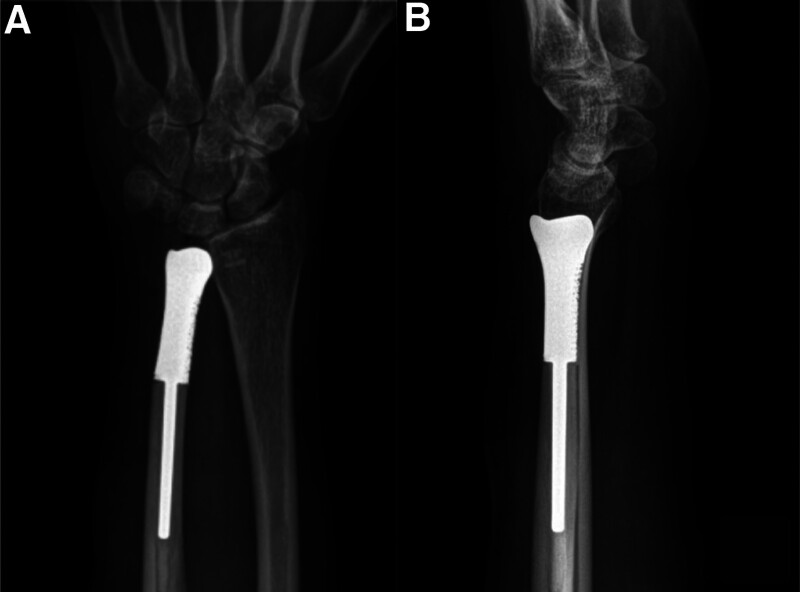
Postoperative radiographs at 1-year follow-up.
